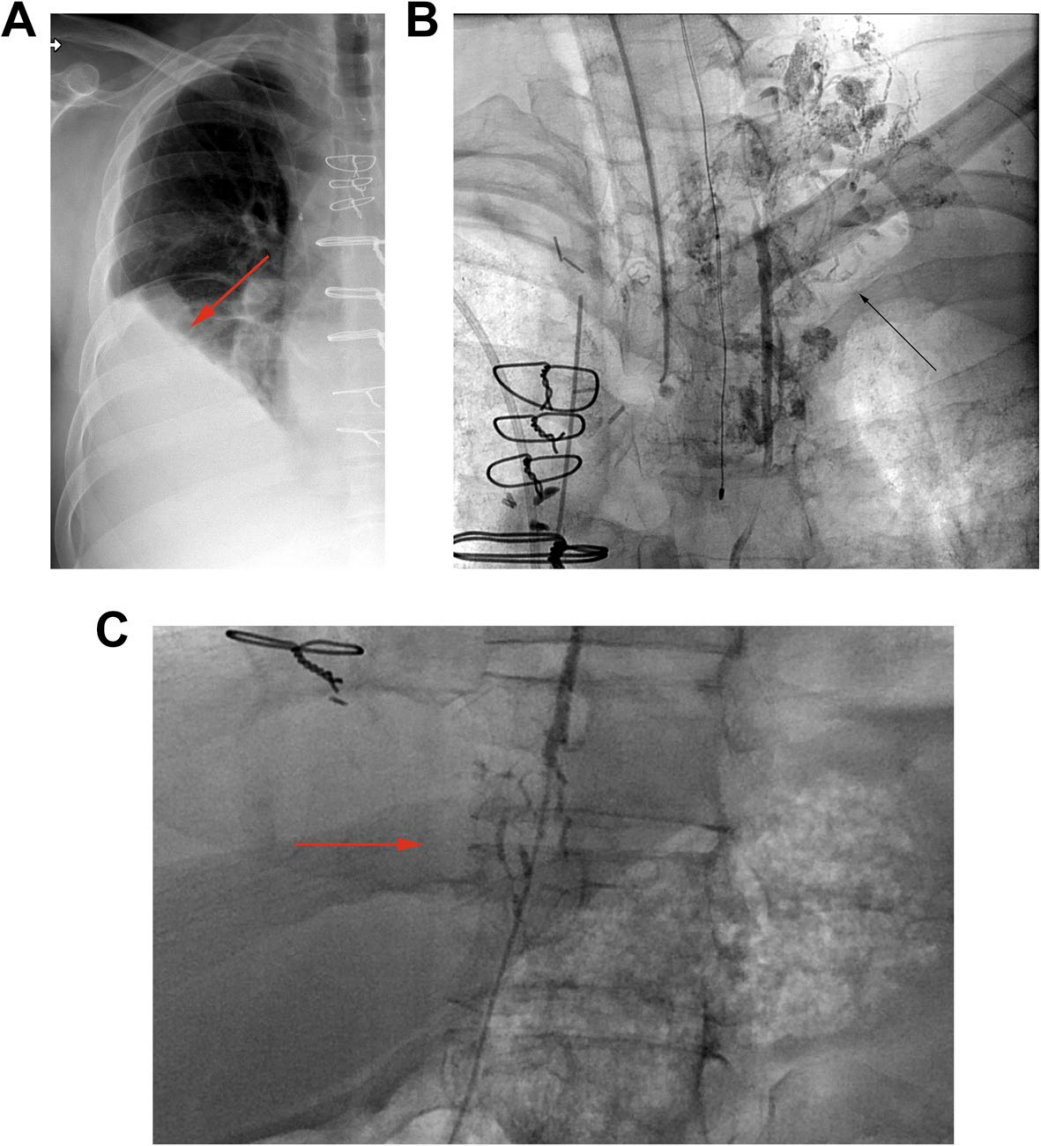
36-year-old male who underwent bilateral lung transplants for cystic fibrosis who developed chylothorax 3 weeks after surgery. A Frontal chest radiograph obtained for worsened dyspnea and hypoxia shows a moderate sized (arrow), increased from previous exams (not shown) and greater than expected for the postoperative time frame. Pleural fluid analysis demonstrated milky fluid with elevated white blood cell and triglyceride levels consistent with chylothorax. B, C Fluoroscopic angiographic images from lymphangiography performed by the interventional radiology service due to persistent chylous effusion despite surgical thoracic duct ligation and talc pleurodesis. There is extravasation of contrast at the level of the mid thoracic duct (arrow in B) and at the level of the diaphragm (arrow in C) indicating chyle leak. The leak resolved after thoracic duct embolization
